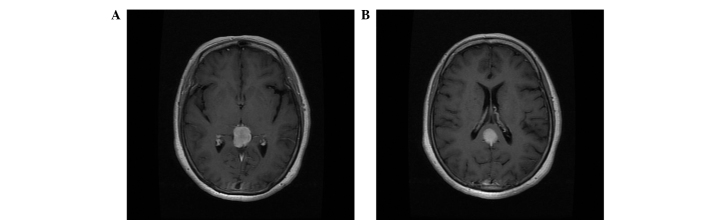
Axial magnetic resonance images demonstrating a well-circumscribed space-occupying lesion in the pineal region. (A) T1-weighted axial MRI of a well-circumscribed solid pineal tumor. (B) T1-weigthed axial MRI of a tumor and the ministry of corpus collosum. MRI, magnetic resonance imaging.
